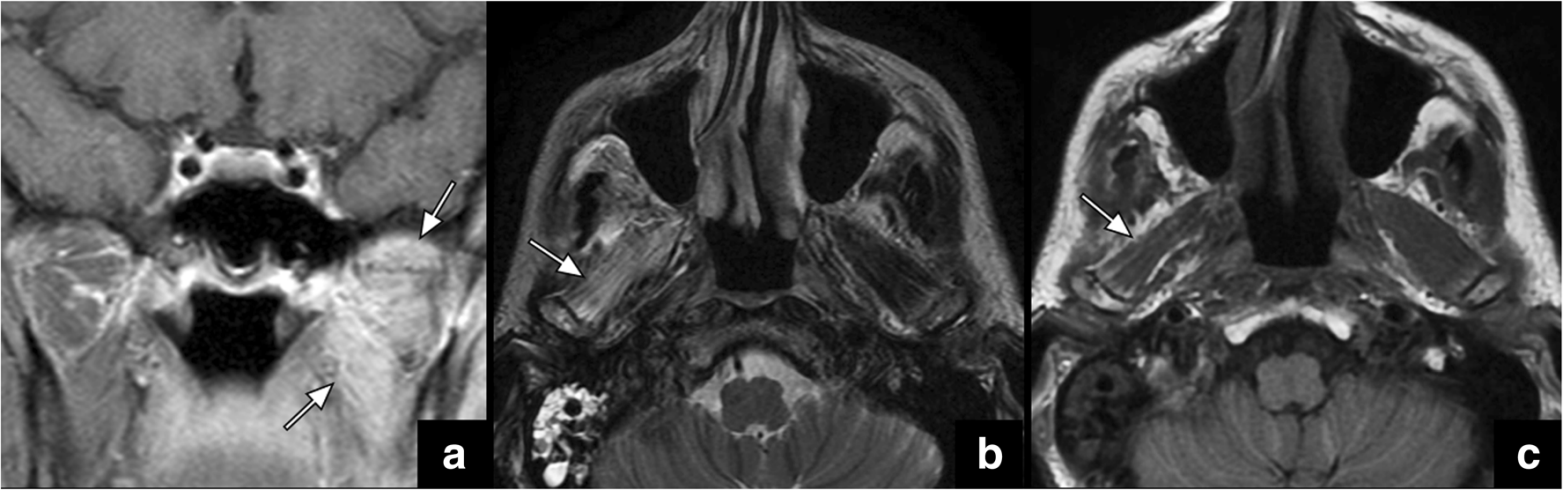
Coronal contrast-enhanced T1-weighted image (a) shows marked enhancement of left pterygoid muscles (*between arrows* in a) indicative of acute denervation of the muscles supplied by the motoric branch of V3. On an axial T2 weighted image in a different patient (b), the denervated muscles show marked hyperintensity (*arrow* in b) due to oedema in the acute phase and due to fatty replacement in the chronic phase. Muscular volume loss is usually the only distinguishing feature between the two on T2-weighted images with atrophy present in the chronic phase. Muscular atrophy and fatty replacement, as reflection of chronic denervation, can also be easily identified on axial T1 sequence (*arrow* in c). These findings can indicate the presence of (motoric) V3 malfunction due to PNTS
